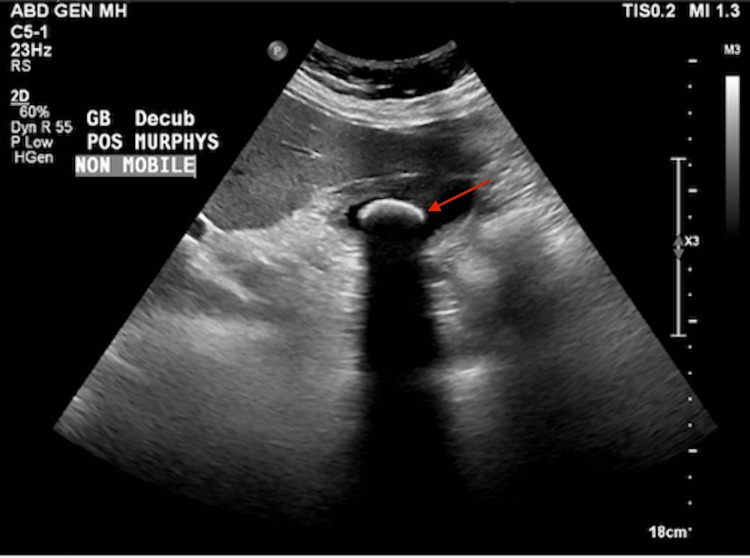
A liver ultrasound showed a non-mobile stone measuring up to 2.7 cm. No evidence of gallbladder wall thickening or pericholecystic fluid. CBD measures 5 mm.
